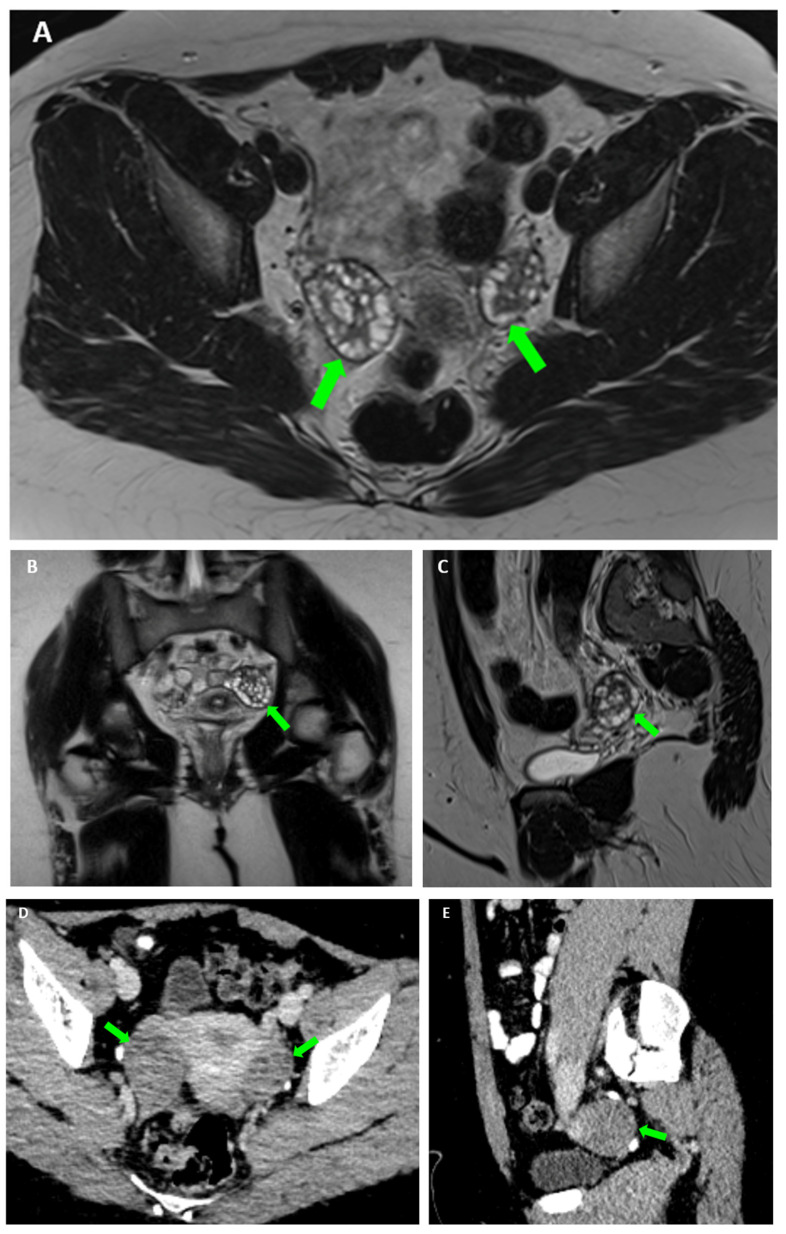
The medical history included spontaneous menarche since the age of 13, irregular menses (3 to 5 cycles per year), in association with obesity and acne. She was diagnosed with polycystic ovary syndrome type A and follow-up during teenager years as outpatient, undergoing a normal puberty with an adult height according to the mid-parental height. Over the years, no particular long-term medication was administered and the weight excess was apparently resistant to usual hypocaloric diets. Within the months before the tumor detection, no distinct clinical clue was retrospectively identified, neither a change in the phenotype. At the timing of adrenalectomy, the body mass index was 37 kg/sqm and no overt Cushing’s syndrome was pointed out. The medical records with respect to the biochemical and hormonal assays revealed at the age of 19 (a few months before the tumor removal): insulin resistance based on a Homeostatic Model Assessment of Insulin Resistance (HOMA-IR) of 4 (Normal < 2) without diabetes/prediabetes; increased dehydroepiandrosterone sulphate (DHEA-S) of 604 (Normal: 148–407) µg/dL and elevated total testosterone level of 5.42 (Normal < 1.67) nmol/L, with normal thyroid function and prolactin [(A–C). Pre-adrenalectomy aspect of the ovaries (green arrows) at MRI: right ovary of 3.2 by 3.9 by 3.3 cm and left ovary of 2.7 by 3.6 by 4.1 cm (both with inhomogeneous structure and multiple peripherally distributed microcysts); (A). native phase, T2 WI FSE, axial plane; (B). native phase, T2 WI HASTE, coronal plane (left ovary); (C). native phase, T2 WI FSE, sagittal plane (left ovary). (D,E). Post-adrenalectomy aspect of the ovaries (green arrow) at CT scan: right ovary of 3.3 by 4.0 by 3.8 cm and left ovary of 2.7 by 5.1 by 4.7 cm, both with a micro-polycystic structure; (D). delayed phase, axial plane; (E). delayed phase, sagittal plane]. After unilateral adrenalectomy, the blood pressure remained normal and the patient did not experience any adrenal insufficiency. Hormonal panel showed a normal adrenocorticotropic hormone (ACTH) of 16.03 (Normal: 7.2–63.3) pg/mL, plasma morning cortisol of 11.5 (Normal: 6.2–19.4) µg/dL, DHEA-S of 90.1 (Normal: 148–407) µg/dL, total testosterone of 0.66 (Normal < 1.67) nmol/L, follicle-stimulant hormone (FSH) of 2.25 mUI/mL, luteinizing hormone (LH) of 6.64 mUI/mL, and estradiol of 40.83 pg/mL (in amenorrhea). A 75g oral glucose tolerance test (OGTT) ruled out an impaired glucose profile based on fasting glycemia of 86 mg/dL, at one hour of 136 mg/dL, and at two hours of 107 mg/dL, while glycated hemoglobin A1c remained normal of 5.2 (Normal: 4.8–5.9) %, as found pre-operatory. Fasting insulin (of 13.2 µUI/mL) increased at 126.3 µUI/mL, respectively, at 42.28 µUI/mL at one hour, respectively, at two hours during OGTT. HOMA-IR improved at 2.8, but remained higher than normal (<2).
